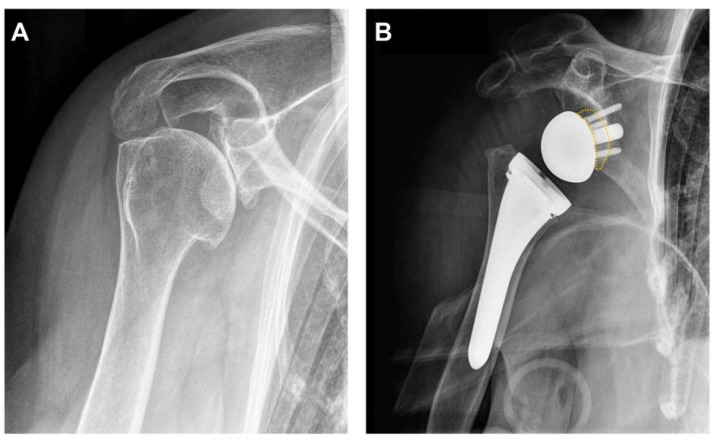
Standard anteroposterior radiographs of a right shoulder before and after implantation of a reverse total shoulder arthroplasty: (A) Cuff arthropathy of the shoulder with increased posterior glenoid wear. (B) Implantation of an uncemented MyShoulder (R) (Medacta, Castel San Pietro, Switzerland). Additionally, a bony lateral increased-offset harvested from the humeral head was implanted between the glenoid baseplate and the native glenoid (yellow dotted line).
